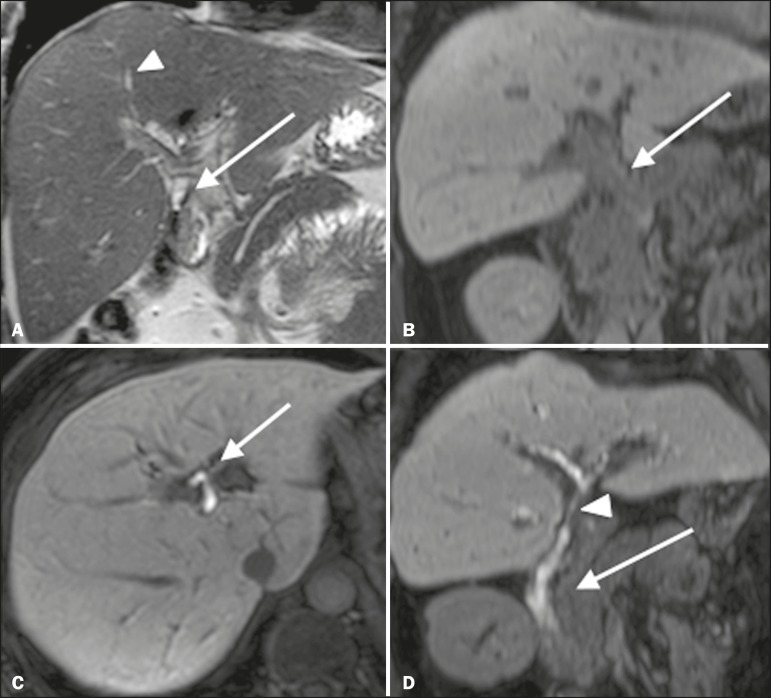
Evaluation of biliaryenteric anastomosis (after complicated cholecystectomy). Coronal T2-weighted sequence (A), and T1- weighted fMRC sequences—axial (C) and coronal (B,D): biliary-enteric anastomosis and mild dilatation of the intrahepatic bile duct 10 min after injection of the contrast medium (arrow and arrowhead, respectively, in A), and delayed excretion of contrast (B), which was not observed until 20 min after injection of the contrast medium (C,D). Patent stenosis of the biliary-enteric anastomosis with delayed biliary excretion.
